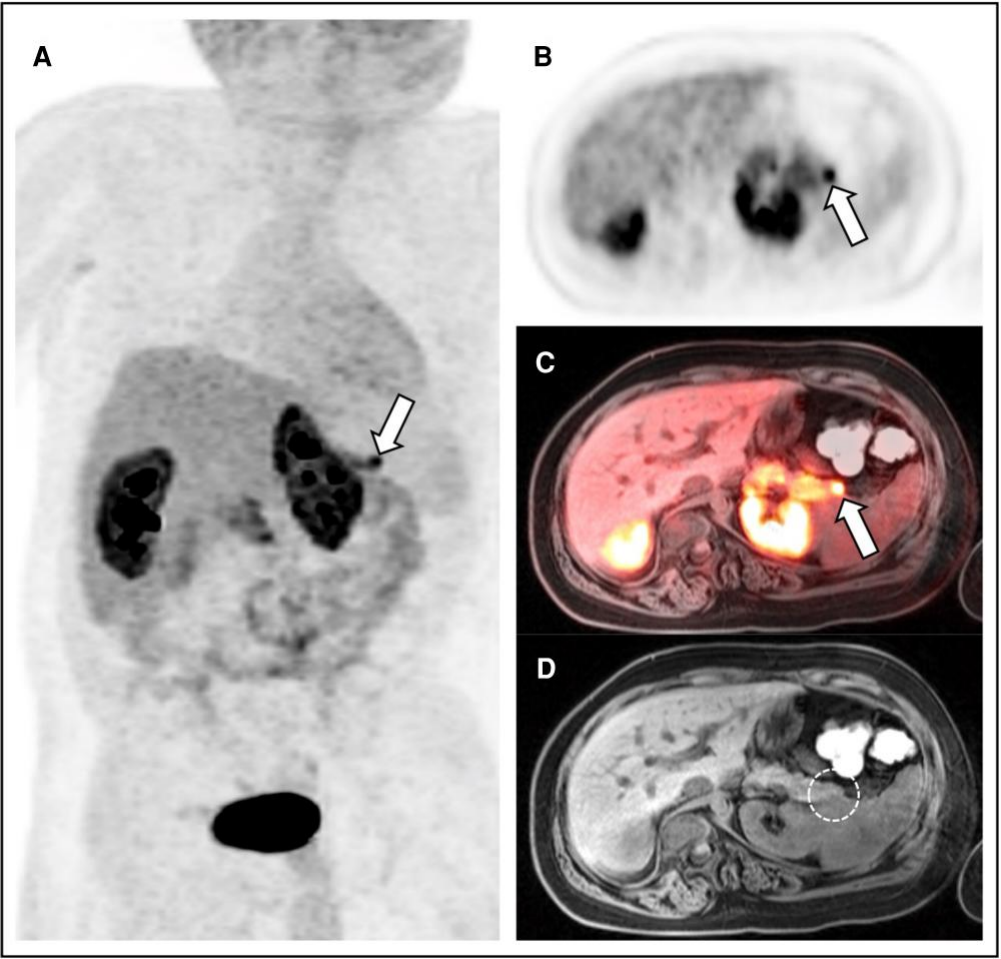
Coronal maximum-intensity-projection (MIP) A, ^18^F-DOPA positron emission tomography (PET); B, axial ^18^F-DOPA PET; C, fused axial ^18^F-DOPA PET/magnetic resonance imaging (MRI); and D, axial noncontrast T1-weighted MRI show a focal intensely tracer-avid lesion (arrows) localizing to the tip of the pancreatic tail but without an anatomic correlate (circle).
